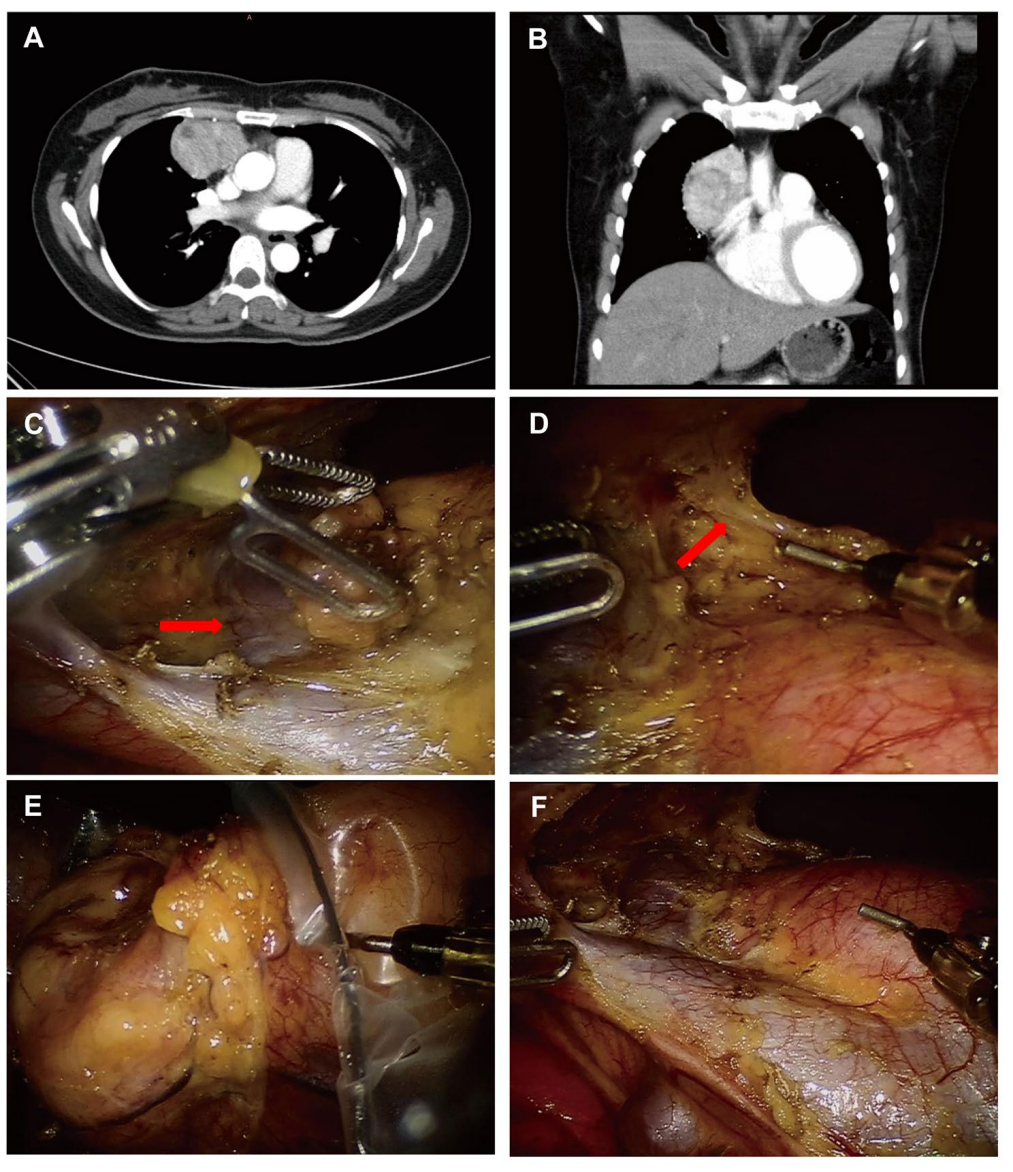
Robot-assisted thymectomy via right-side thoracic approach for a patient with large thymoma. (A) and (B) Preoperative computed tomographic scan of a 37-year old woman with a suspected thymoma (tumor size 4.1 × 5.3 × 6.5 cm). (C) The left innominate vein (red arrow) and superior vena cava were exposed clearly. (D) Intraoperative right thoracoscopic view of the left phrenic nerve (red arrow). (E) The thymoma was removed using a retrieval bag. (F) Surgical field after complete resection of the tumor
